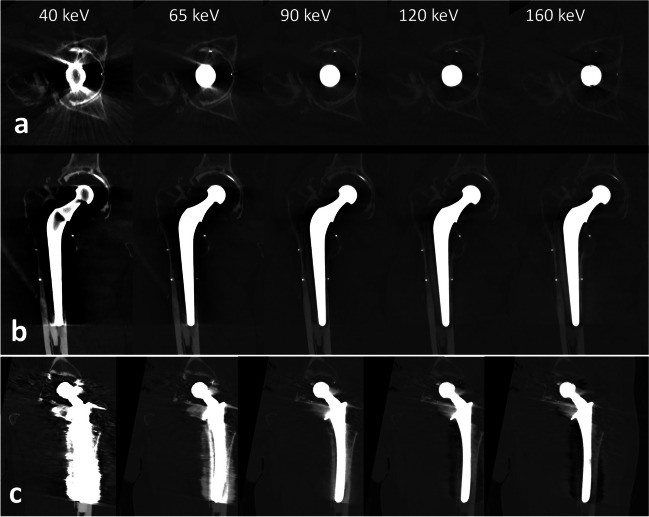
Total hip replacement imaging with PCD-CT virtual monoenergetic images: a transverse and (b) coronal reformats of a right total hip replacement and coronal reformats of a left total joint replacement (c), with increasing energy levels. The acquisition was performed at 140 kVp, with soft tissue kernel and no iterative metal artifact reduction. PCD-CT generates virtual monoenergetic images that range from 40 to 160 keV that progressively reduces metal artifacts around total hip replacements, allowing for improved evaluation of the metal-bone interface and surrounding structures. Note that in those clinical cases, the “sweet spot” of optimal metal artifact reduction is around 90 keV, with some degree of degradation in image quality at higher keV
